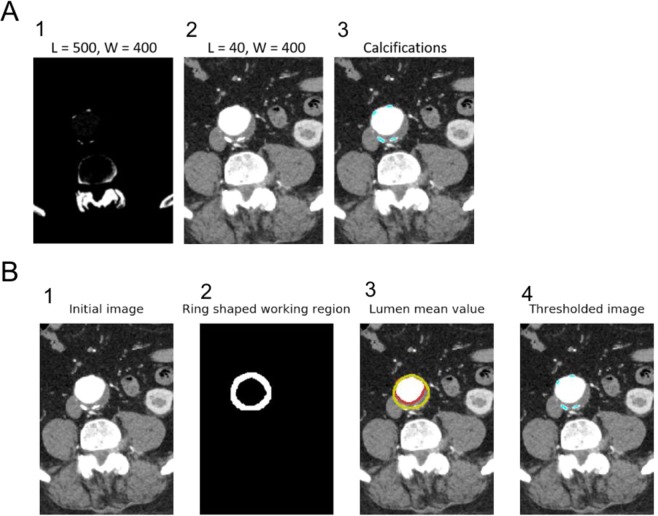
Segmentation of calcifications. (A) Visualization of calcified tissues and contrast medium in CT-scan images using LUT algorithm. L corresponds to grey level; W corresponds to the window (range of values of Hounsfield units converted into grey levels). (B) Steps to discriminate calcifications in the aortic lumen and the intraluminal thrombus.
